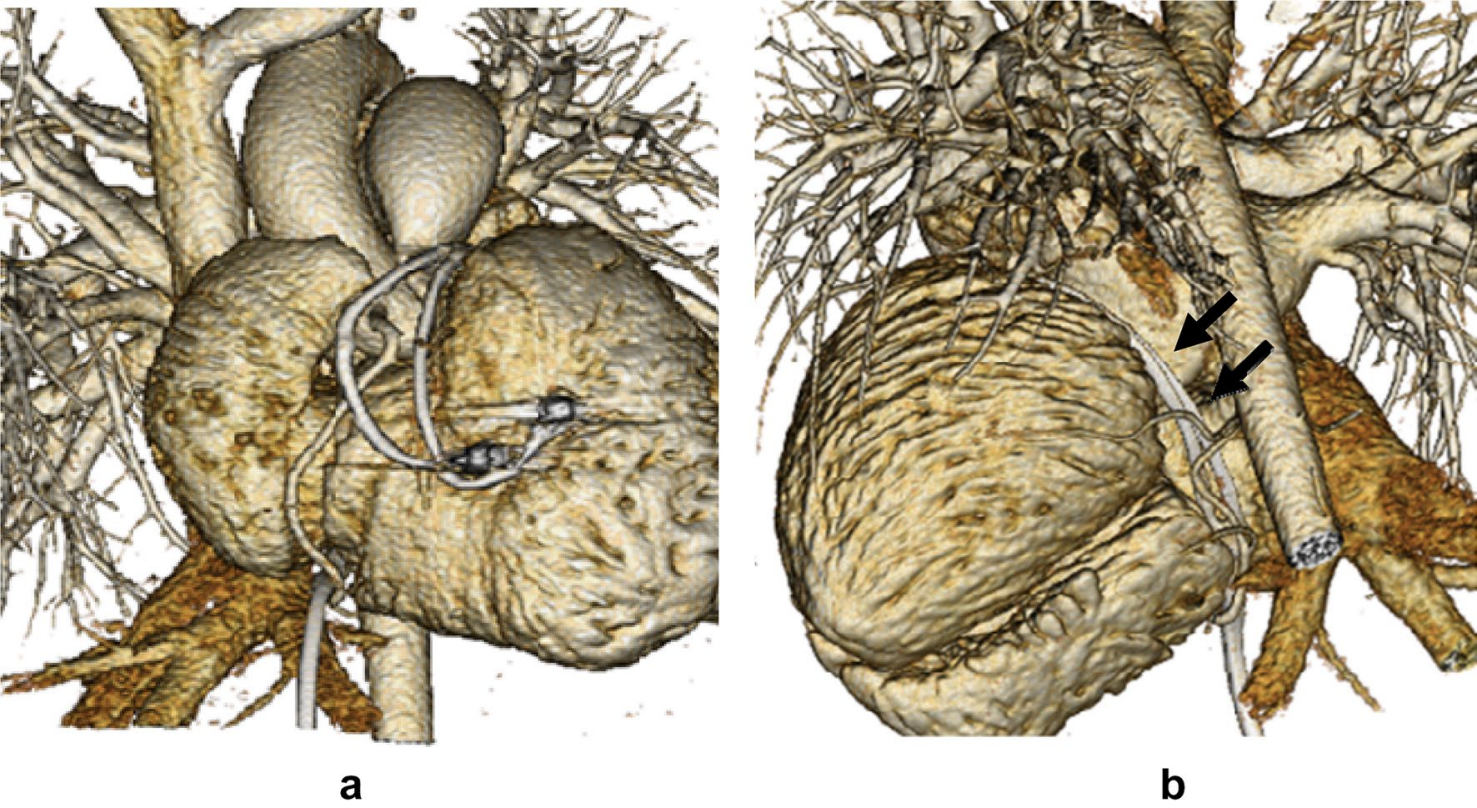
Three-dimensional computed tomography images. a The frontal view shows the epicardial leads compressing the main pulmonary artery. b The lateral view shows the leads extending down along the atrioventricular groove (arrows)
